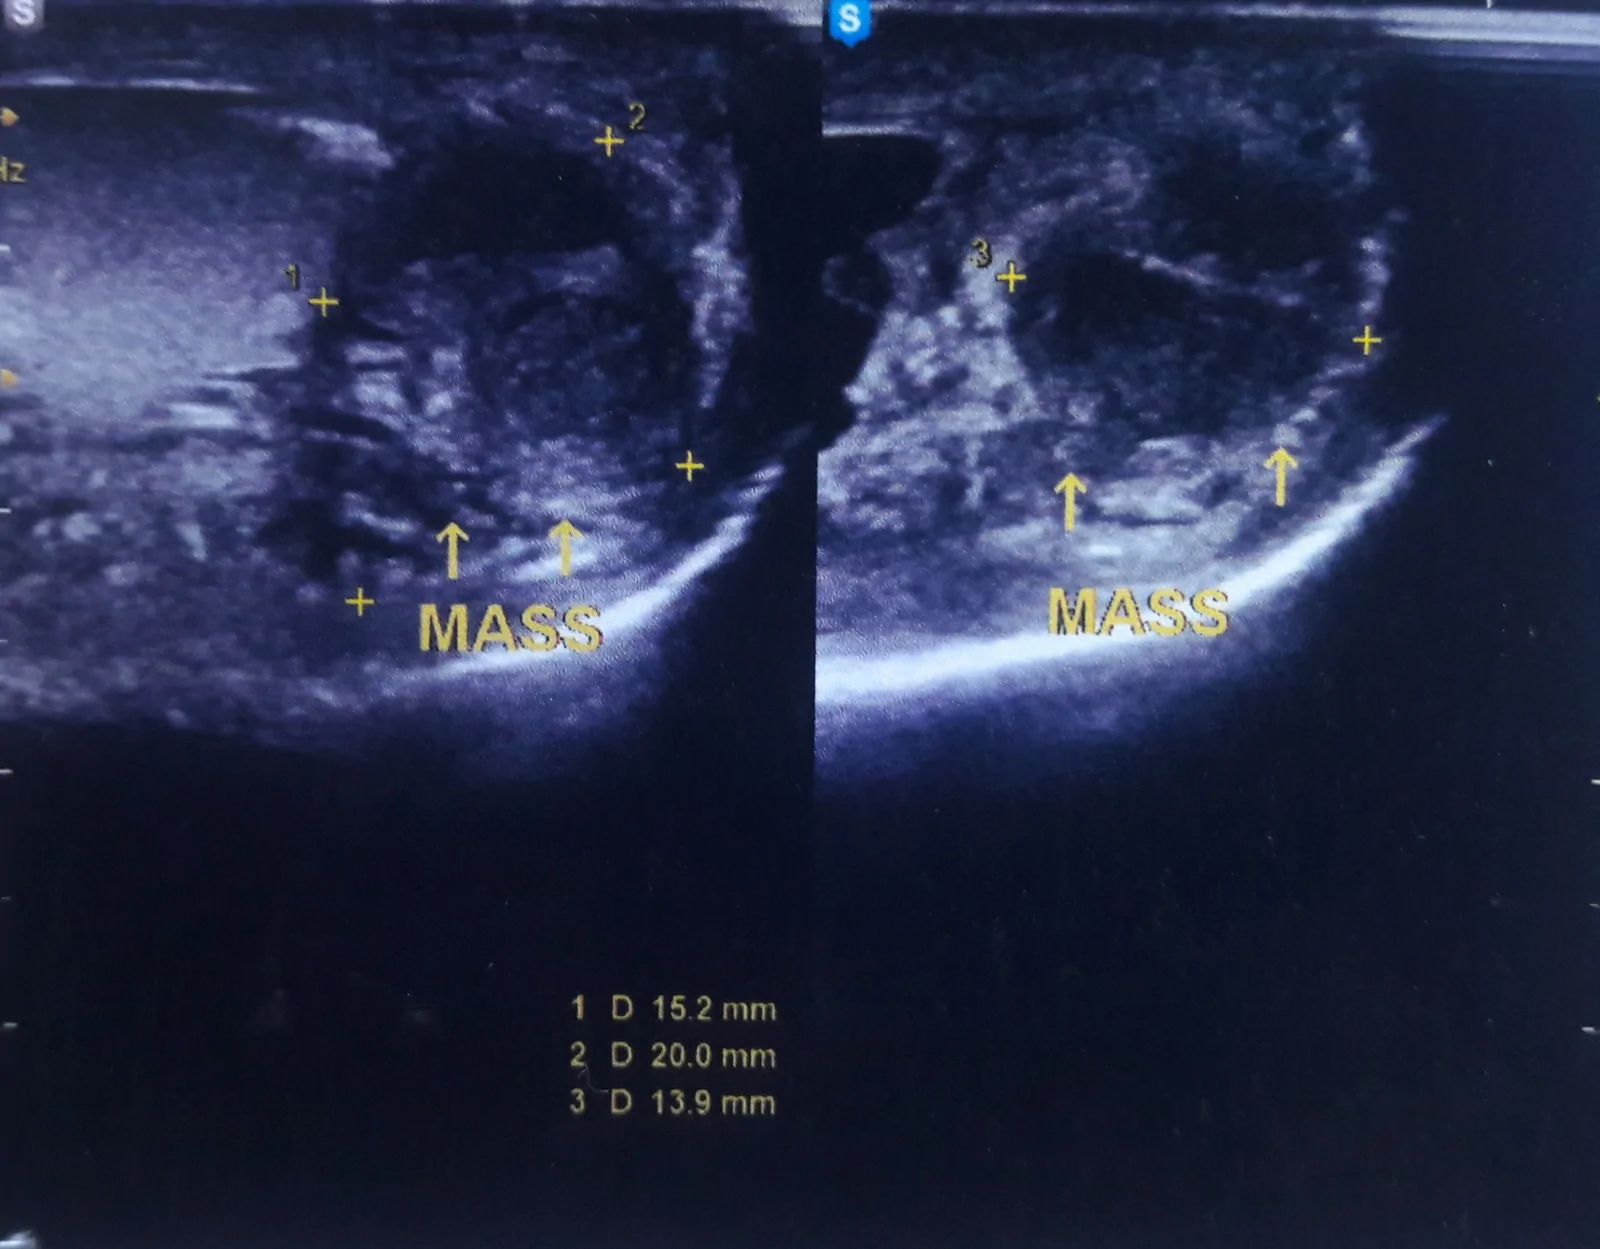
Scrotal ultrasound showing mass near inferior part of left epididymis of case 1. Arrows are directing towards mass (significant lesion) and plus sign is measuring the area of mass.
